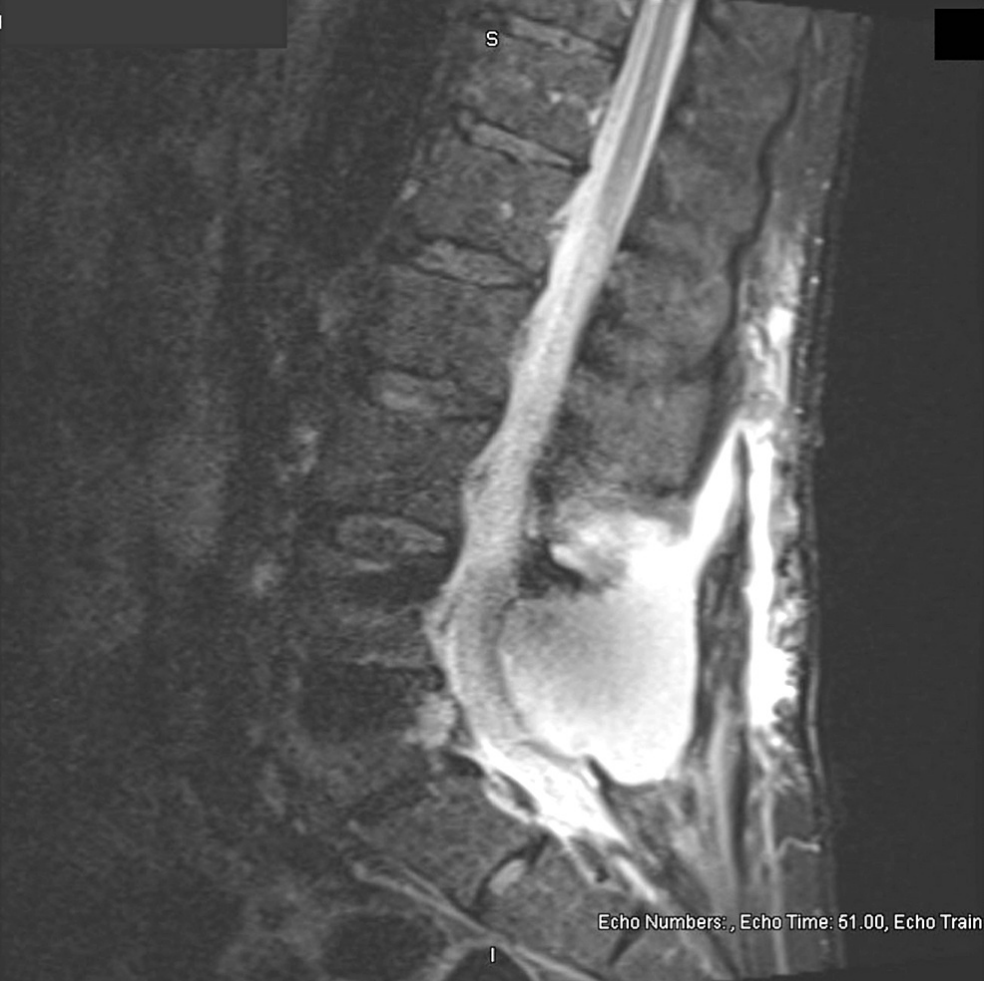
Large posterior mass with fistulous tract visible on sagittal lumbosacral T2-weighted MRI without contrast. Mass measures 5x8x9-cm (AP, transverse, and vertical, respectively).
